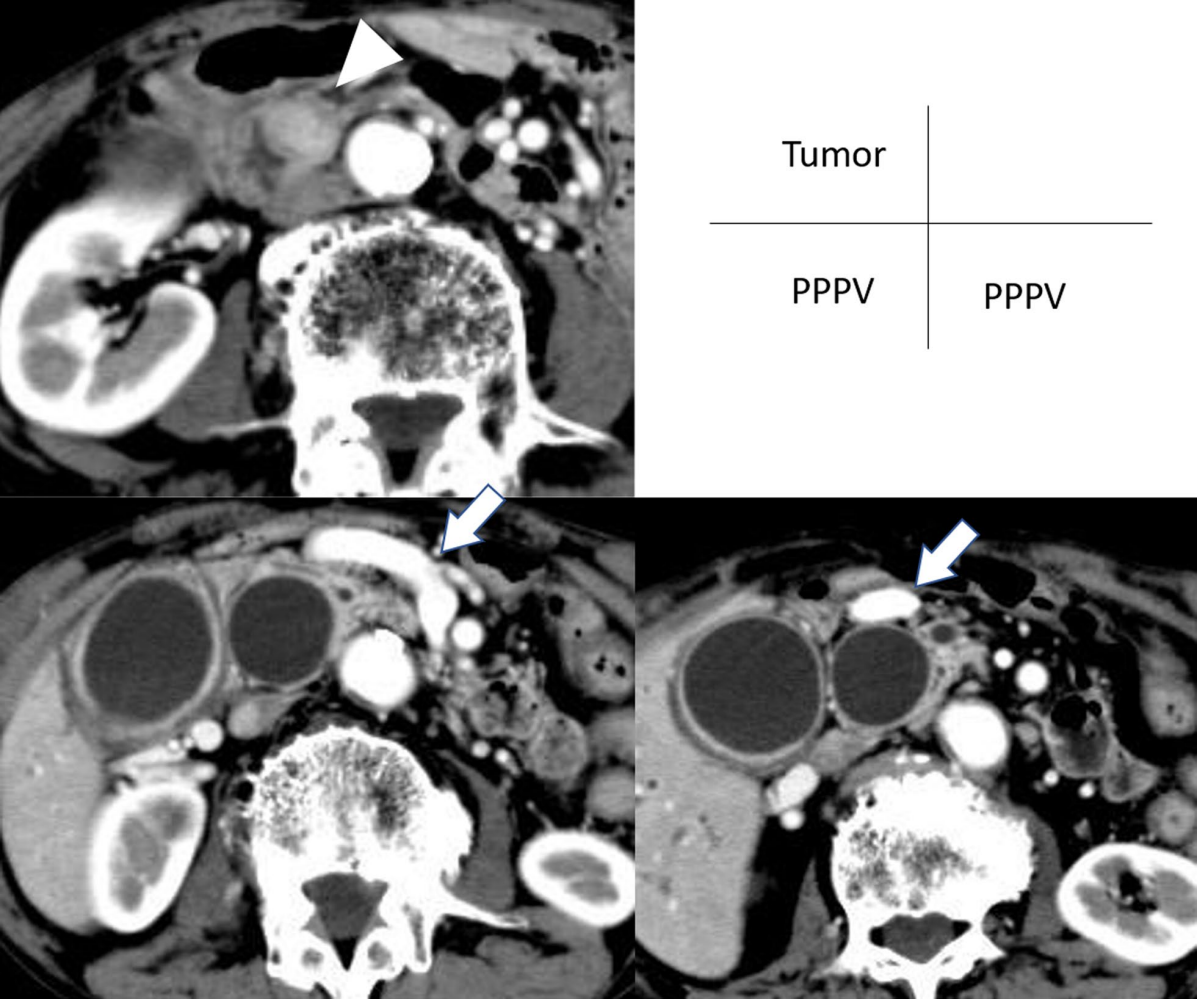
Contrast-enhanced CT image. A A tumor in the ampulla of Vater with low contrast was detected (arrowhead). B A PPPV (arrow) were divaricated from the SMV and crossed in front of the pancreas. C The PPPV crossed in front of the dilated common bile duct
